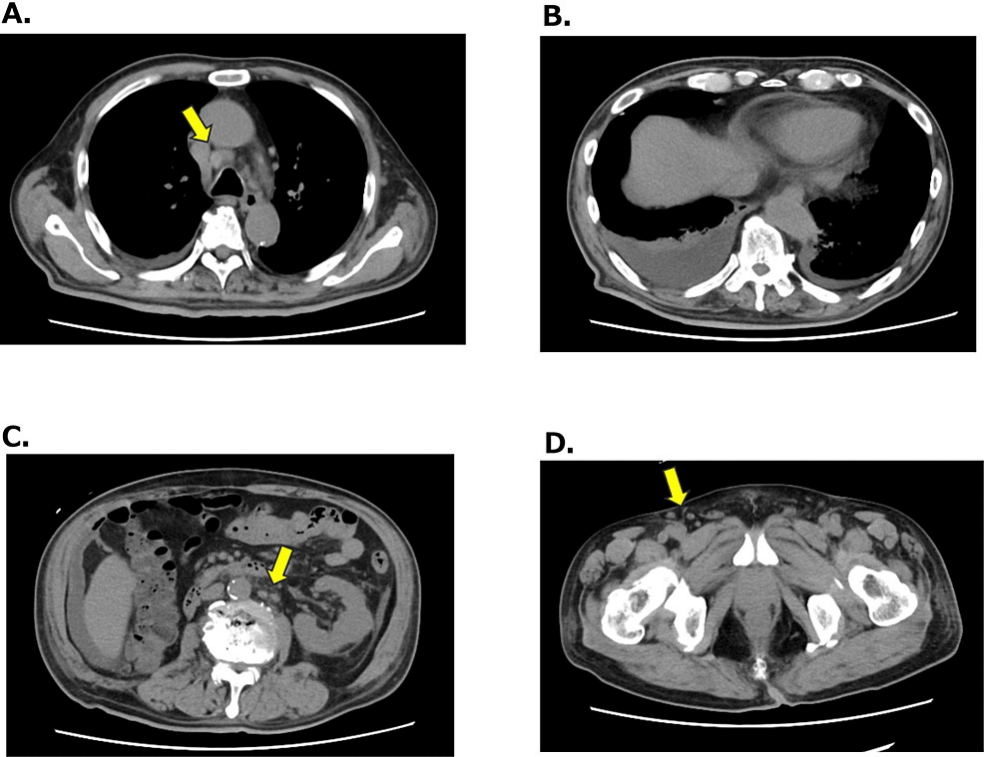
CT on admission Chest CT scan showed mediastinal lymph node swelling (A) and bilateral pleural effusion (B). The abdominal CT image demonstrated ascites and paraaortic lymph node swelling, as well as post nephrectomy (C), and lymph node swelling in the right inguinal fossa (D) CT: computed tomography
